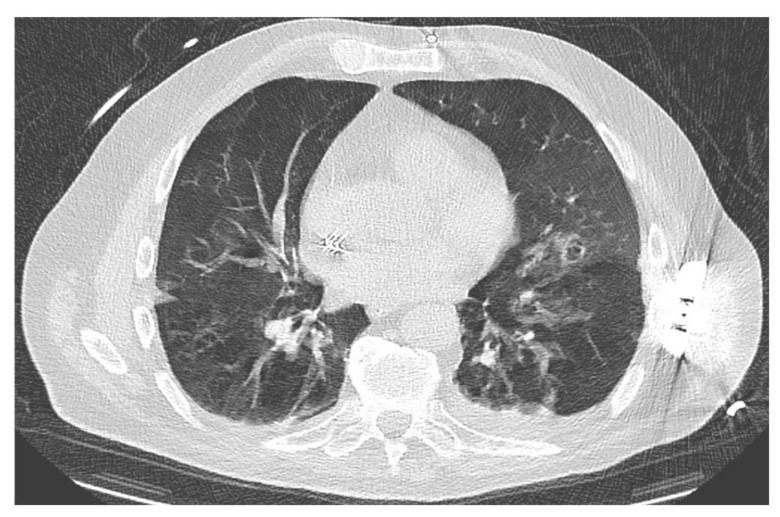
CMV pneumonia. CT scan with diffuse bronchocentric pulmonary ground-glass opacities predominantly in the left lower and left upper lobe.
